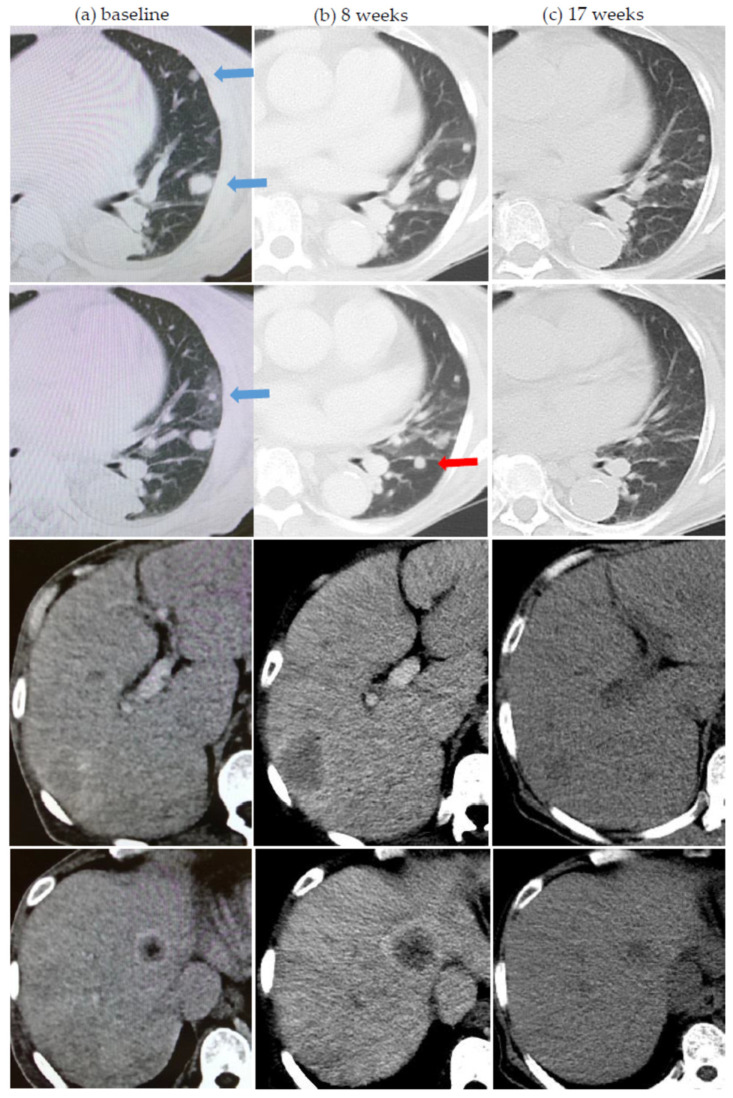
Two atypical response patterns in a 73 year-old lady on first-line nivolumab for metastatic renal cell carcinoma. (a) Baseline computer tomography (CT) scan showing lung and liver metastases before starting single-agent nivolumab. (b) First assessment CT scan after 8 weeks showing dissociated response—improvement and stability in lung metastases (blue arrows in baseline scan) but obvious worsening of liver metastases. (c) Second assessment CT scan at 17 weeks showing continued improvement of lung metastases and now also improvement of liver metastases indicating prior pseudoprogression. On further scrutiny, one small lung lesion (red arrow) had also undergone pseudoprogression.
